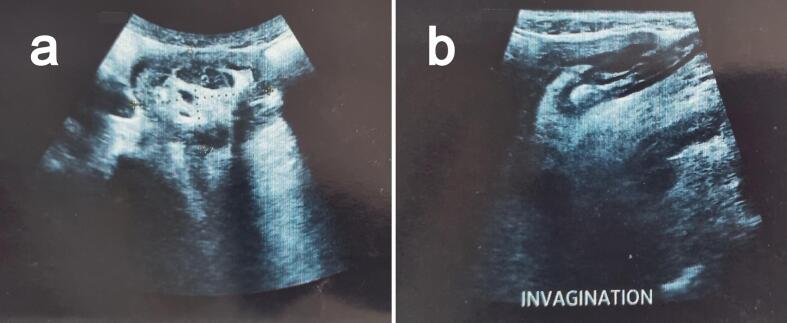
Ultrasonography findings. (a) Transverse view showing the target sign. (b) Longitudinal view showing the pseudo-kidney sign.
